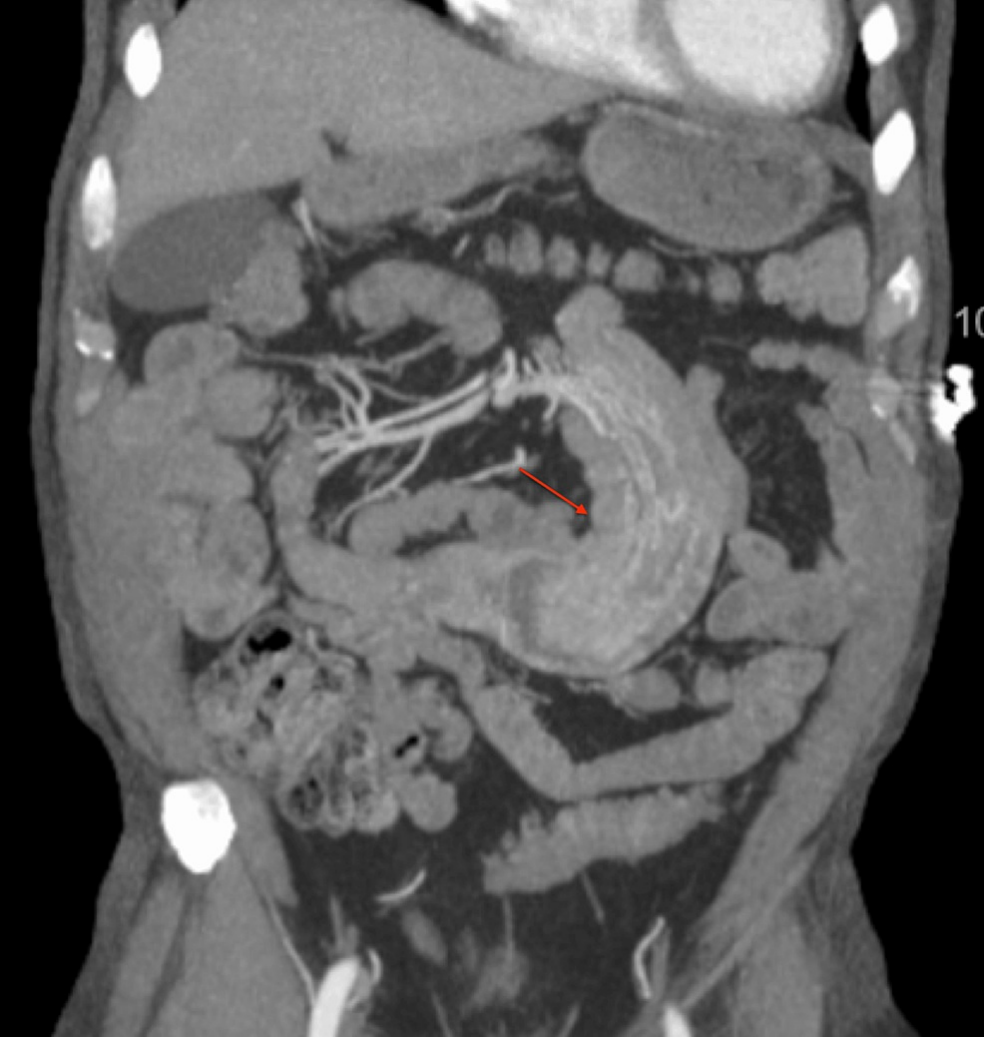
Contrast-enhanced coronal CT image depicting small bowel intussusception CT: computed tomography
